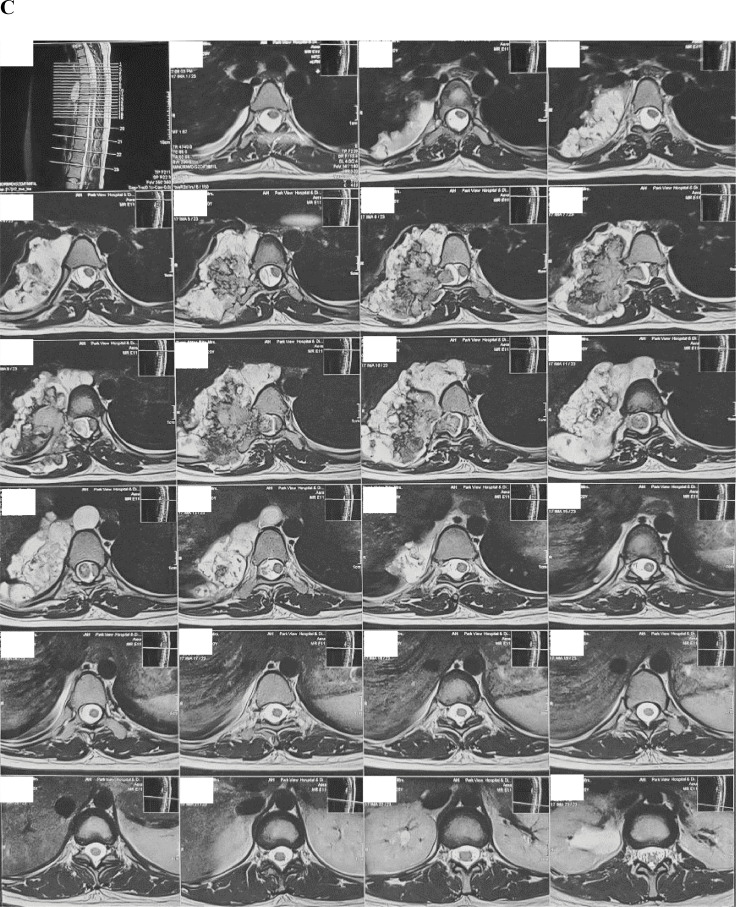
MRI images show a large right paraspinal tumor at level T_6_-T_9_ compressing the spinal cord involving the right parietal pleura. (A) Coronal plane MRI: T2-weighted sequence without gadolinium enhancement, (B) Sagittal plane MRI: T2-weighted sequence without gadolinium enhancement and (C) Axial plane MRI: T2-weighted sequence without gadolinium enhancement.
